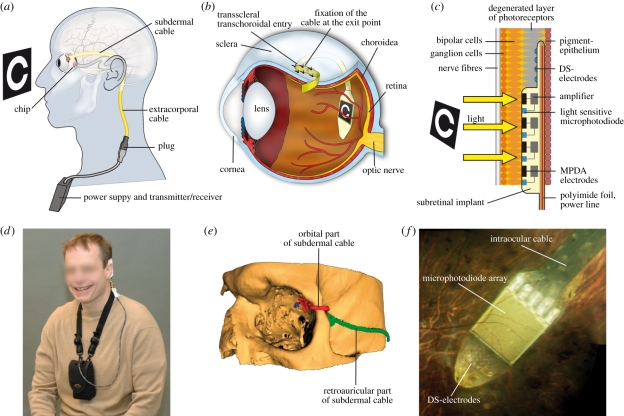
Implant position in the body. (*a*) The cable from the implanted chip in the eye leads under the temporal muscle to the exit behind the ear, and connects with a wirelessly operated power control unit. (*b*) Position of the implant under the transparent retina. (*c*) MPDA photodiodes, amplifiers and electrodes in relation to retinal neurons and pigment epithelium. (*d*) Patient with wireless control unit attached to a neckband. (*e*) Route of the polyimide foil (red) and cable (green) in the orbit in a three-dimensional reconstruction of CT scans. (*f*) Photograph of the subretinal implant's tip at the posterior eye pole through a patient's pupil.
